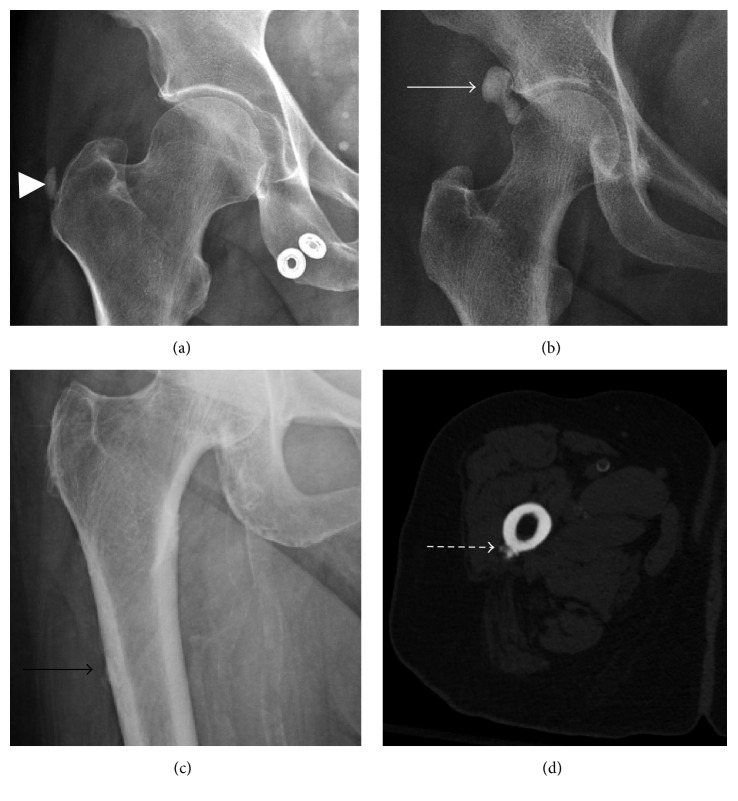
Calcific tendinopathy of the hip. (a) Gluteus medius/minimus calcific tendinopathy (arrow head) is best appreciated on AP hip or pelvis radiographs. (b) Rectus femoris calcific tendinopathy most commonly presents lateral to the acetabular weight-bearing surface (solid arrow). (c) and (d) Calcific tendinopathy of the gluteus maximus tendon. The gluteus maximus tendon calcification almost always involves the tendon insertion on the gluteal tubercle along the posterolateral subtrochanteric femur (black arrow). Cortical erosion and periosteal reaction are frequently seen (dashed arrow), which can easily be mistaken for a juxtacortical malignancy.
